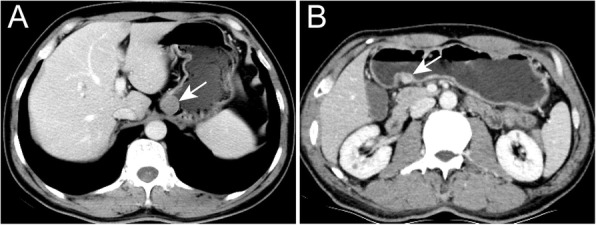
Representative CT images in the venous phase of stromal tumors (white arrows). a The axial image shows a will-defined round mass in the gastric upper body with endo-luminal growth pattern. The LD/SD ratio of this lesion is 1.12 (20.14/17.85 mm). The relative enhancement ratio of HP masses to the pancreas is 0.59 (56.87/96.81 HU). b The axial image shows an ill-defined round mass in the gastric lower body with exo-luminal growth pattern
